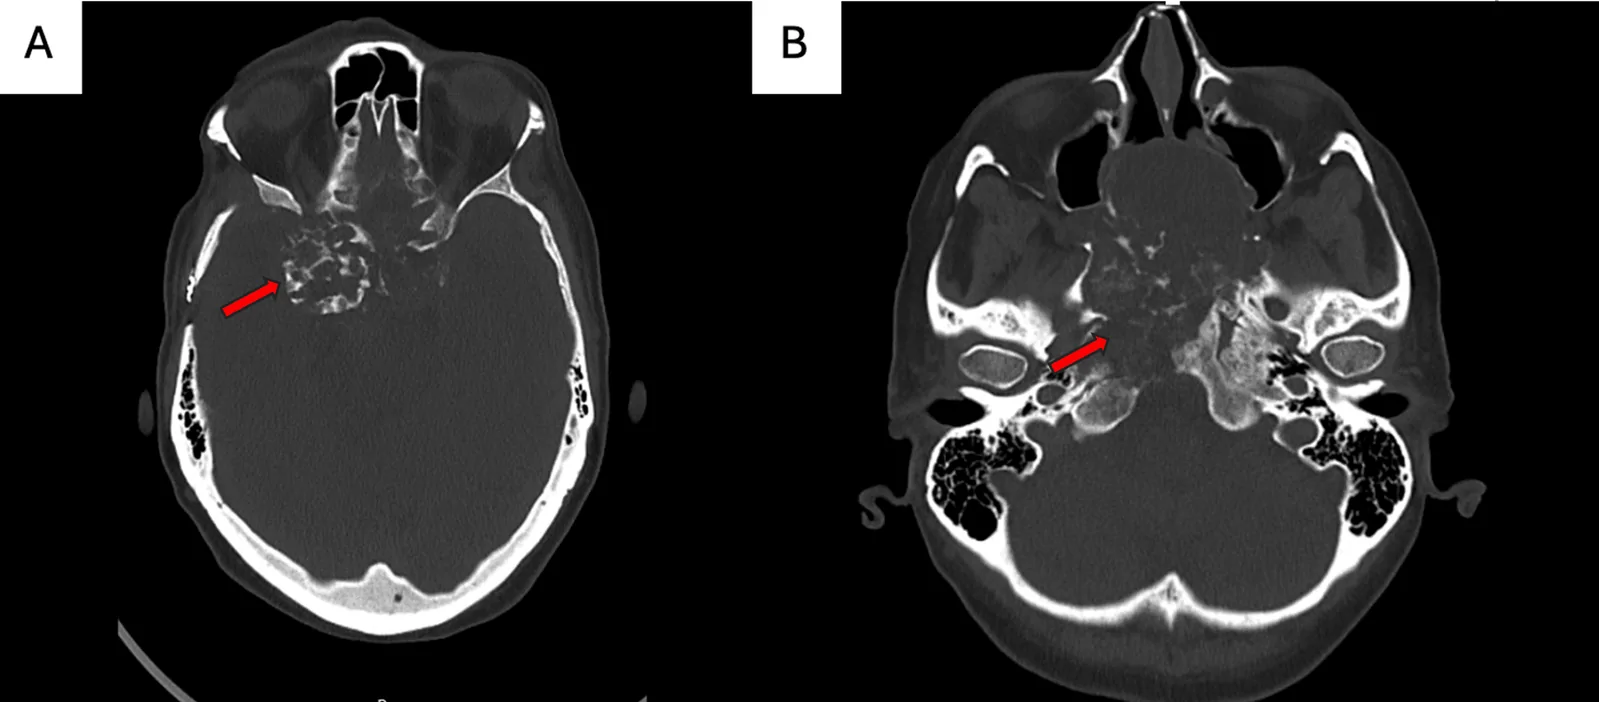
Computed tomography demonstrating osseous destruction of the skull base in May 2025 (A-B) Axial computed tomography images obtained in May 2025 in the bone window demonstrate an expansile, destructive lesion centered in the clivus, with extensive osseous erosion and involvement of the adjacent skull base. Red arrows indicate the areas of osseous involvement.
